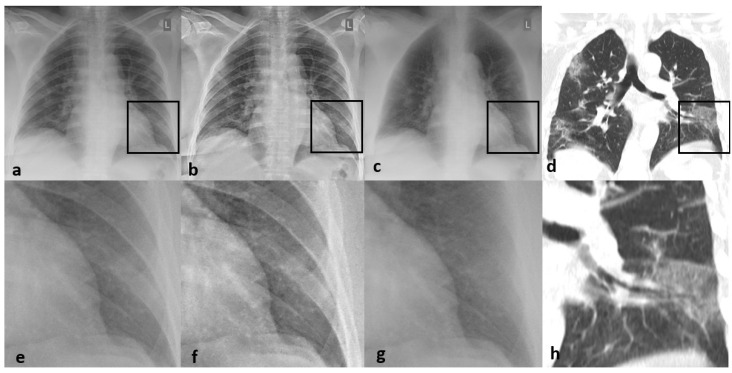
53-year-old male patient with rt-PCR proven COVID-19 pneumonia symptomatic since 6 days with fever and cough showing typical lung changes of COVID-19 pneumonia on (d,h) CT with wedge-shaped subpleural ground-glass opacification (GGO). These changes are hidden by the ribs in the (a,e) conventional radiograph (CRX), slightly visible on the (b,f) enhanced CRX, and better visible on the (c,g) enhanced CRX with bone suppression.
